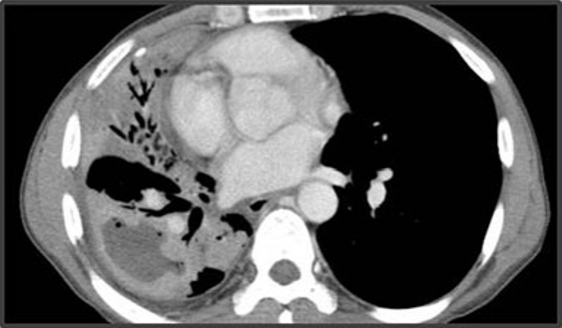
Transfissural extension of parenchymal actinomycosis in a 43-year-old man with high fever for 10 days manifesting as chronic necrotising pneumonia. Chest CT scan in mediastinal window setting shows multifocal lobar consolidation containing a large low attenuation area in the right lower and middle lobe, which demonstrates extension across the fissure
